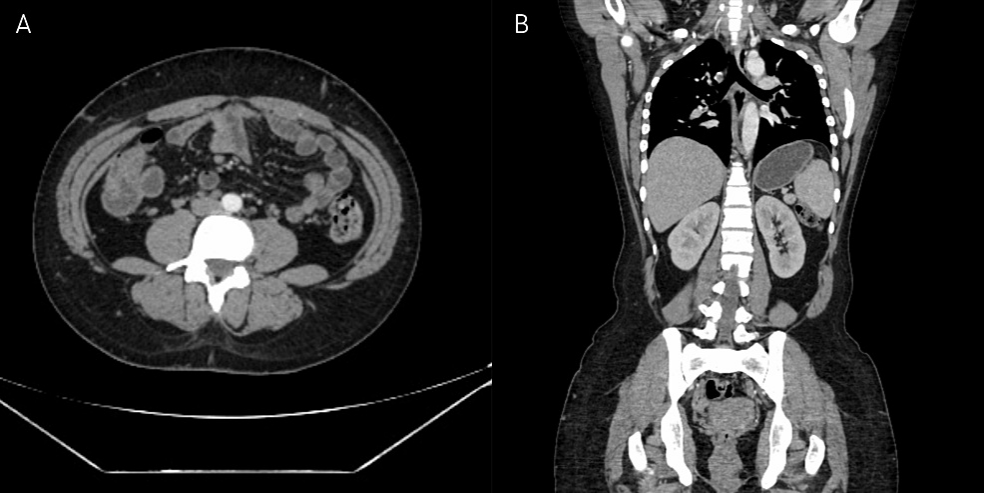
Abdominal CT during follow-up Multiple abdominal CT images: axial view (A), and coronal view (B), demonstrating no evidence of local recurrence or metastasis.
